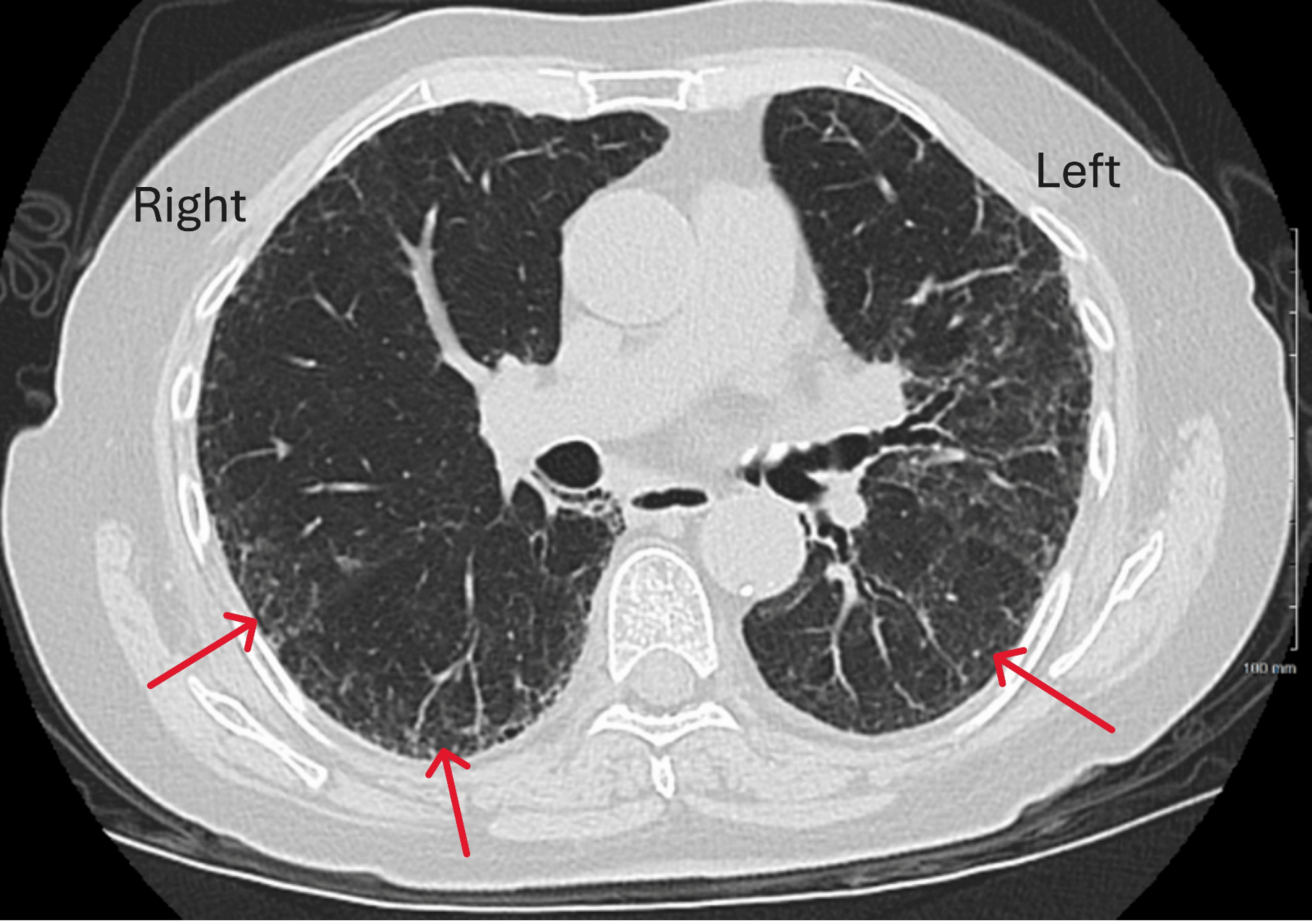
CT chest Significant improvement of pulmonary infiltrates in both lungs in comparison to Figure 1.
